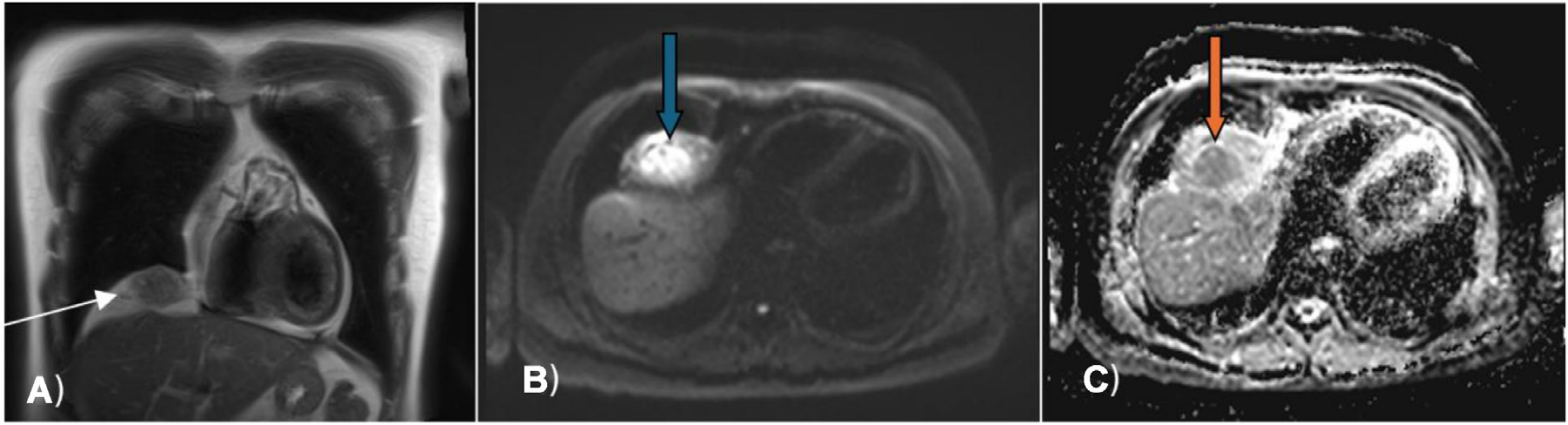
Coronal T2 weighted MR image of the chest (A) demonstrates heterogeneous signal intensity within the mass (white arrow). Blue arrow in the diffusion weighted MR image (B) points to the increased signal intensity within the mass; and corresponding low signal intensity (orange arrow) on the ADC map image (C).
